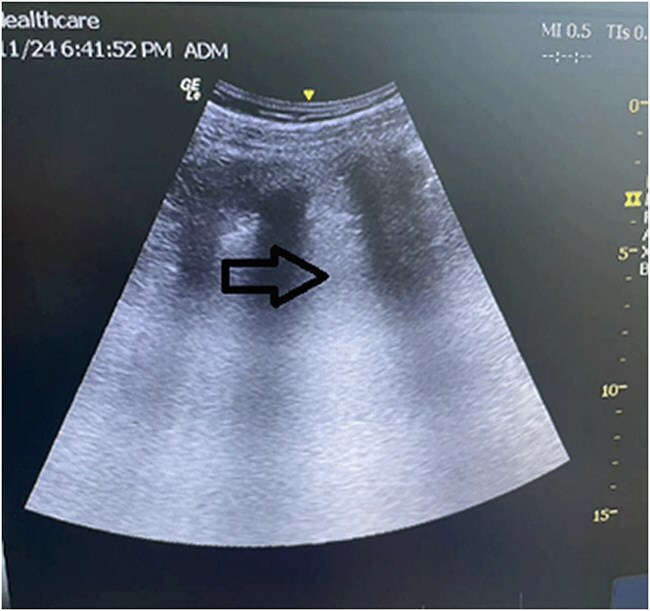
Lung ultrasonography revealed diffuse B-lines consistent with pulmonary edema.
